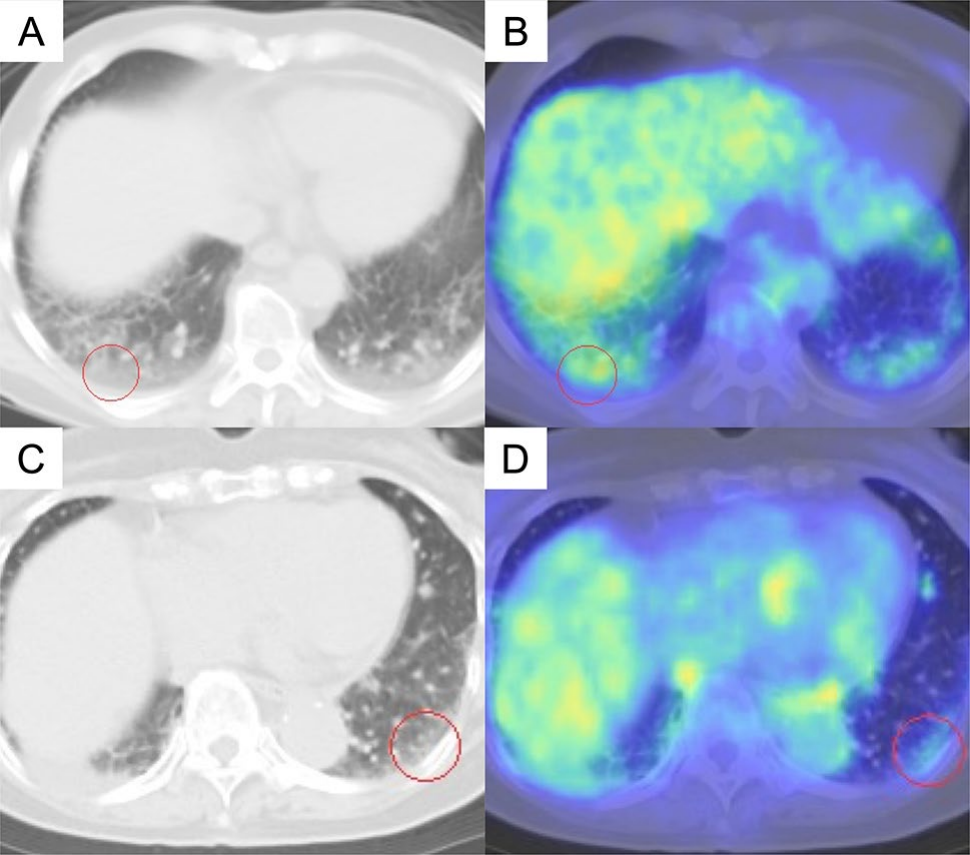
FDG accumulation in the interstitial lesions. Representative cases of strong (A, B) and weak (C, D) FDG accumulation with SUVpeak values of 2.30 and 1.72, respectively, on PET-CT in the interstitial lesions
